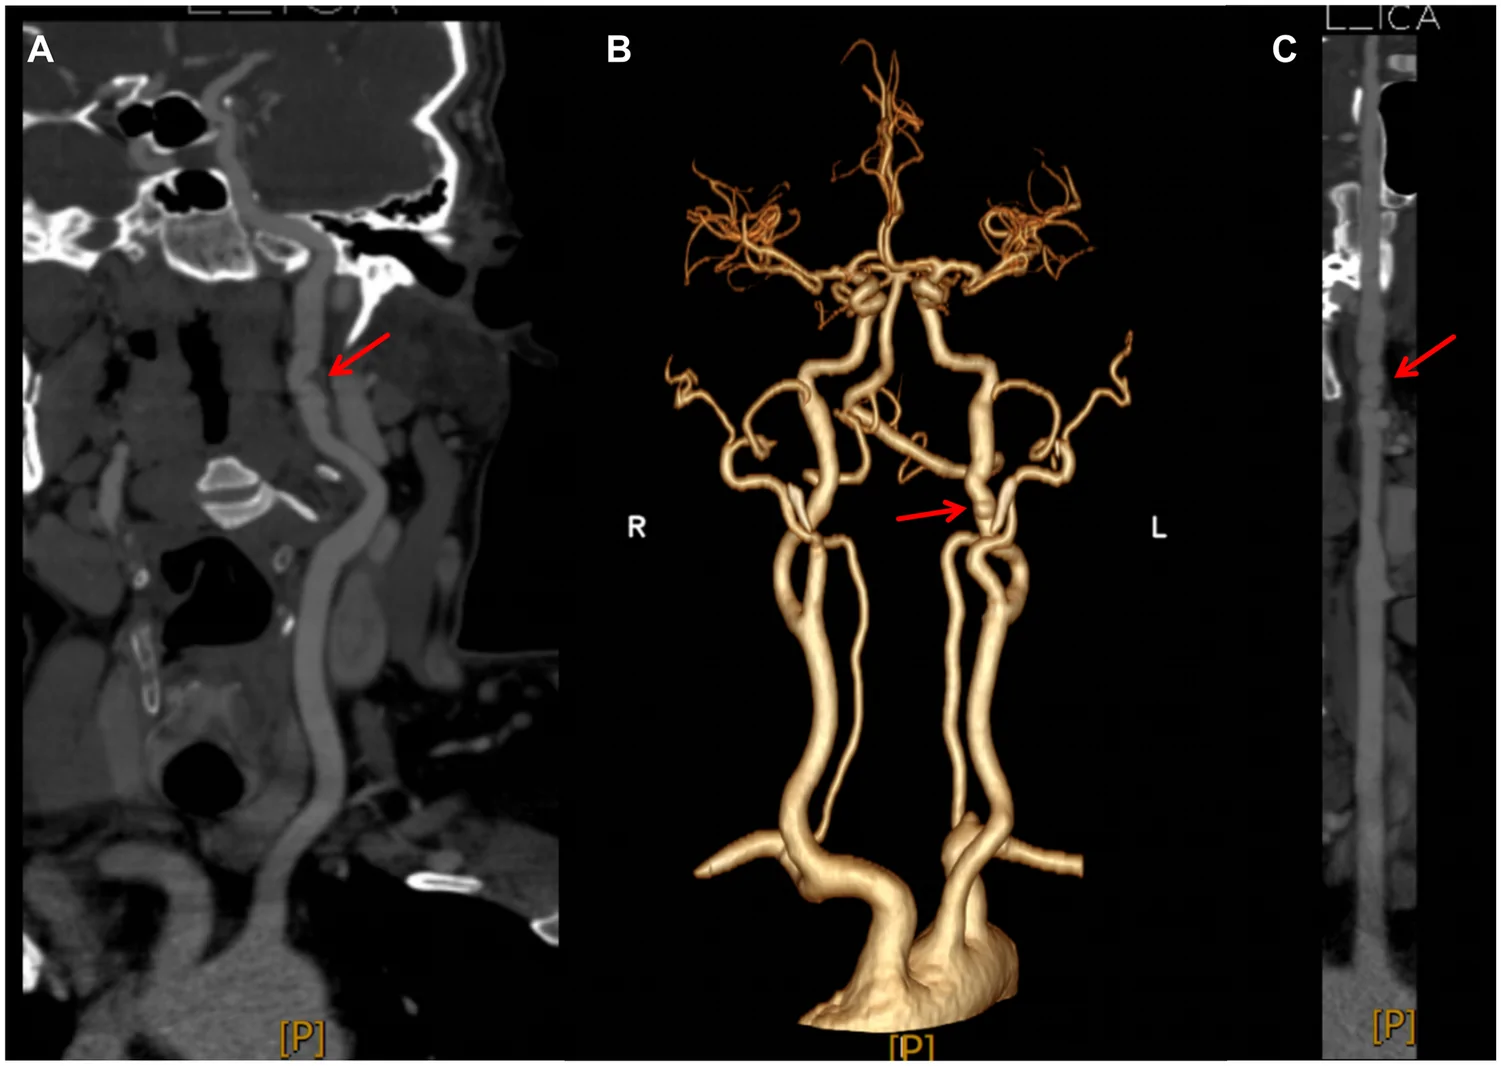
Cervicocerebral computed tomography angiography (CTA) of the left internal carotid artery (L-ICA). (A–C) Multiplanar reconstructions of cervical CTA demonstrate the characteristic “string-of-beads” appearance in the mid-cervical C1 segment of the left internal carotid artery (red arrows), characterized by alternating segments of focal arterial stenosis and post-stenotic dilatation. This typical angiographic finding on CTA is highly suggestive of fibromuscular dysplasia (FMD), and the multi-view imaging clearly depicts the spatial distribution of the abnormal vascular segment without obvious atherosclerotic plaque or vascular dissection in the surrounding vessels.
